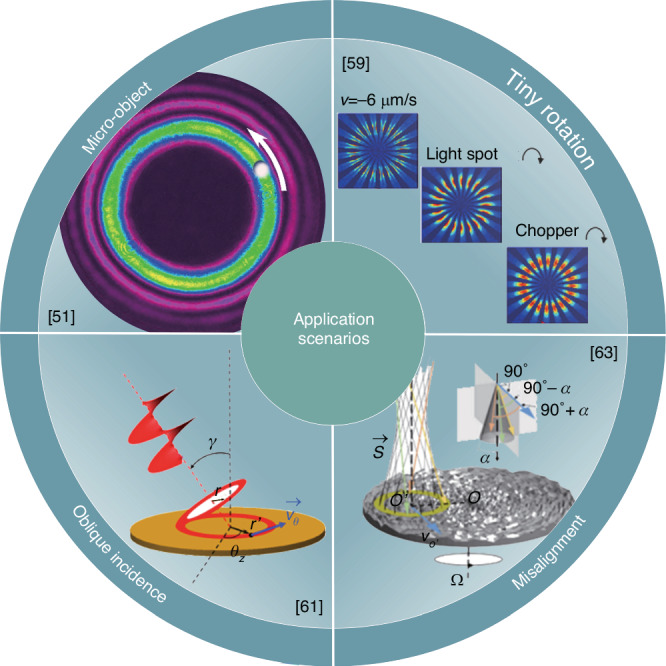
Applications of the rotational Doppler effect (RDE) metrology. RDE metrology with vortex beams exhibits exceptional adaptability across various conditions, ranging from ideal to complex scenarios such as micro-object rotation detection, tiny rotations, oblique incidence, and lateral misalignment. Utilizing vortex beams with opposite-sign OAM in both reference and measurement paths, this approach accurately identifies micro displacements, the direction of micro-object rotation, and minute velocity changes. The central frequency remains stable despite variations in incident angle and lateral displacement, while the rotational Doppler signal broadens, ensuring extraction of rotational velocity even in cases of oblique incidence and lateral misalignment. References given in the insets
